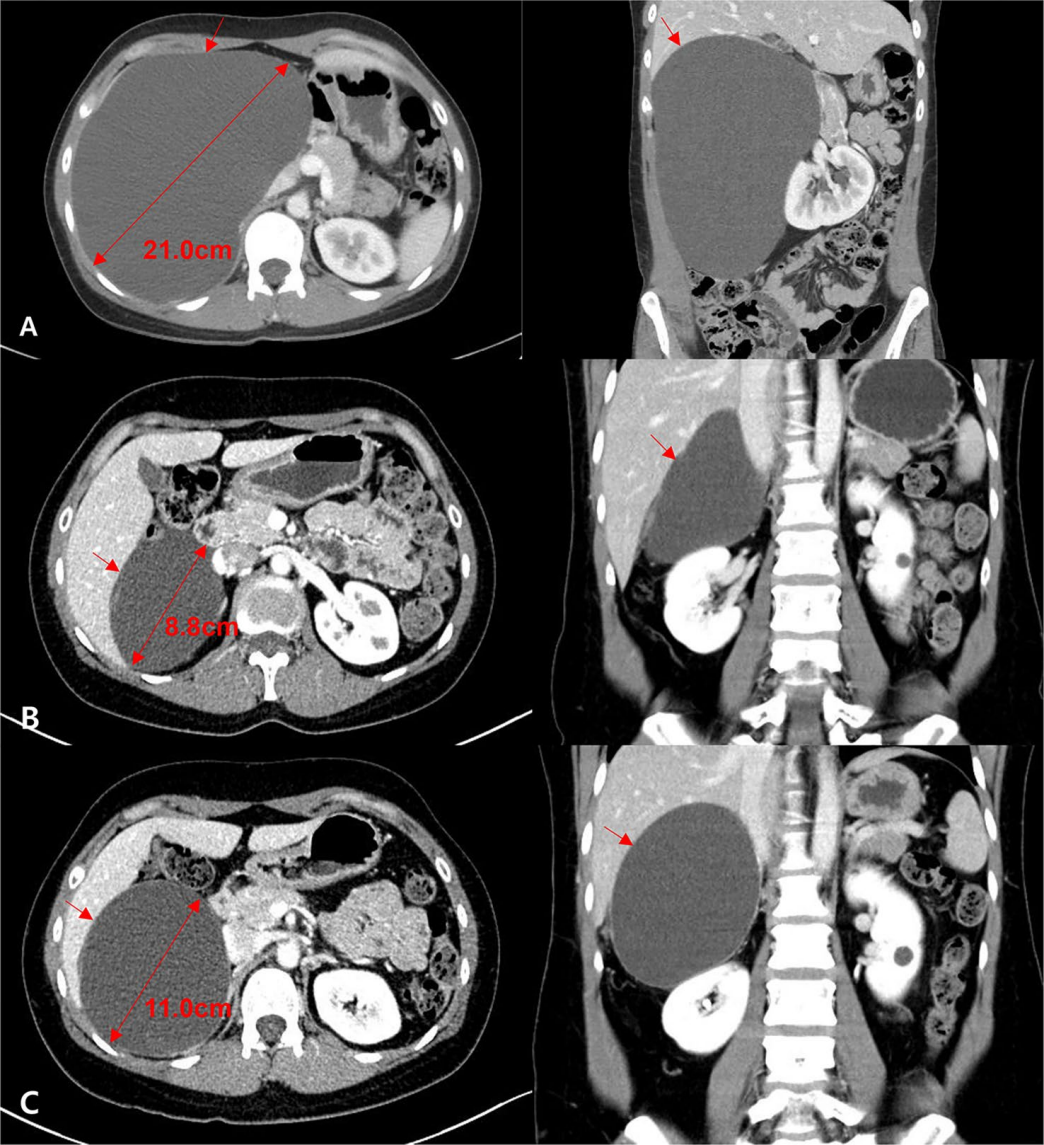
Axial and sagittal computed tomography (CT) imaging of Patient No. 6 (A) prior to treatment, (B) 3 months after RFA treatment, and (C) 12 months after RFA treatment, at the time of cyst recurrence.
